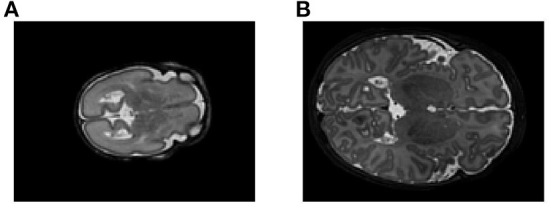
Axial slices of T2-weighted scans of: (A) the youngest subject, 26.71 weeks post-conceptional age, vs. (B) the oldest subject, 45.14 weeks post-conceptional age in the dataset. The head circumference of the oldest infant on the left is 36 cm, compared to 21 cm of the youngest infant on the right.
